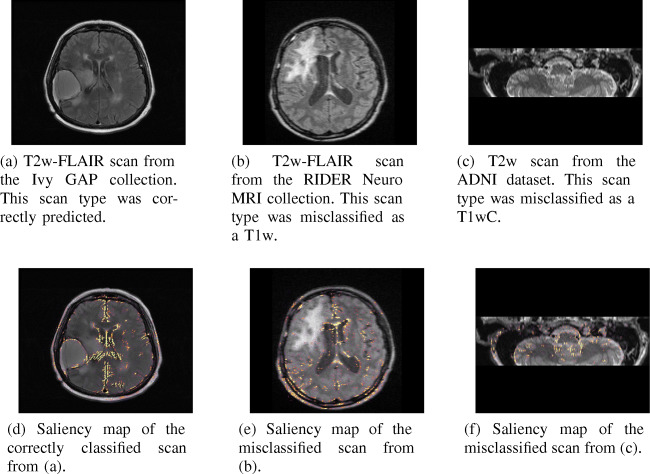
Examples of scans our method misclassified (b and c) and a correctly classified scan (a) as comparison, along with their saliency maps. The T2w-FLAIR scan in (b) is probably misclassified as its appearance is very different from T2w-FLAIR scans that were in the train dataset. The T2w scan in (c) is probably misclassified because it has a very limited field of view
